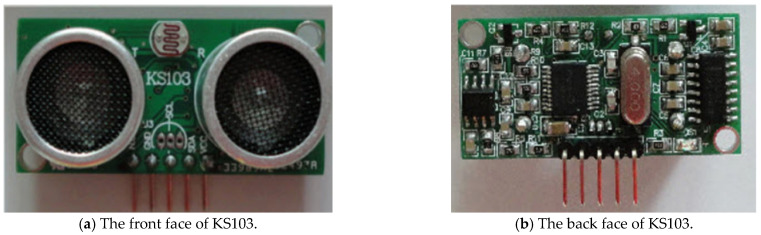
Photo of ultrasonic sensor module KS103.
